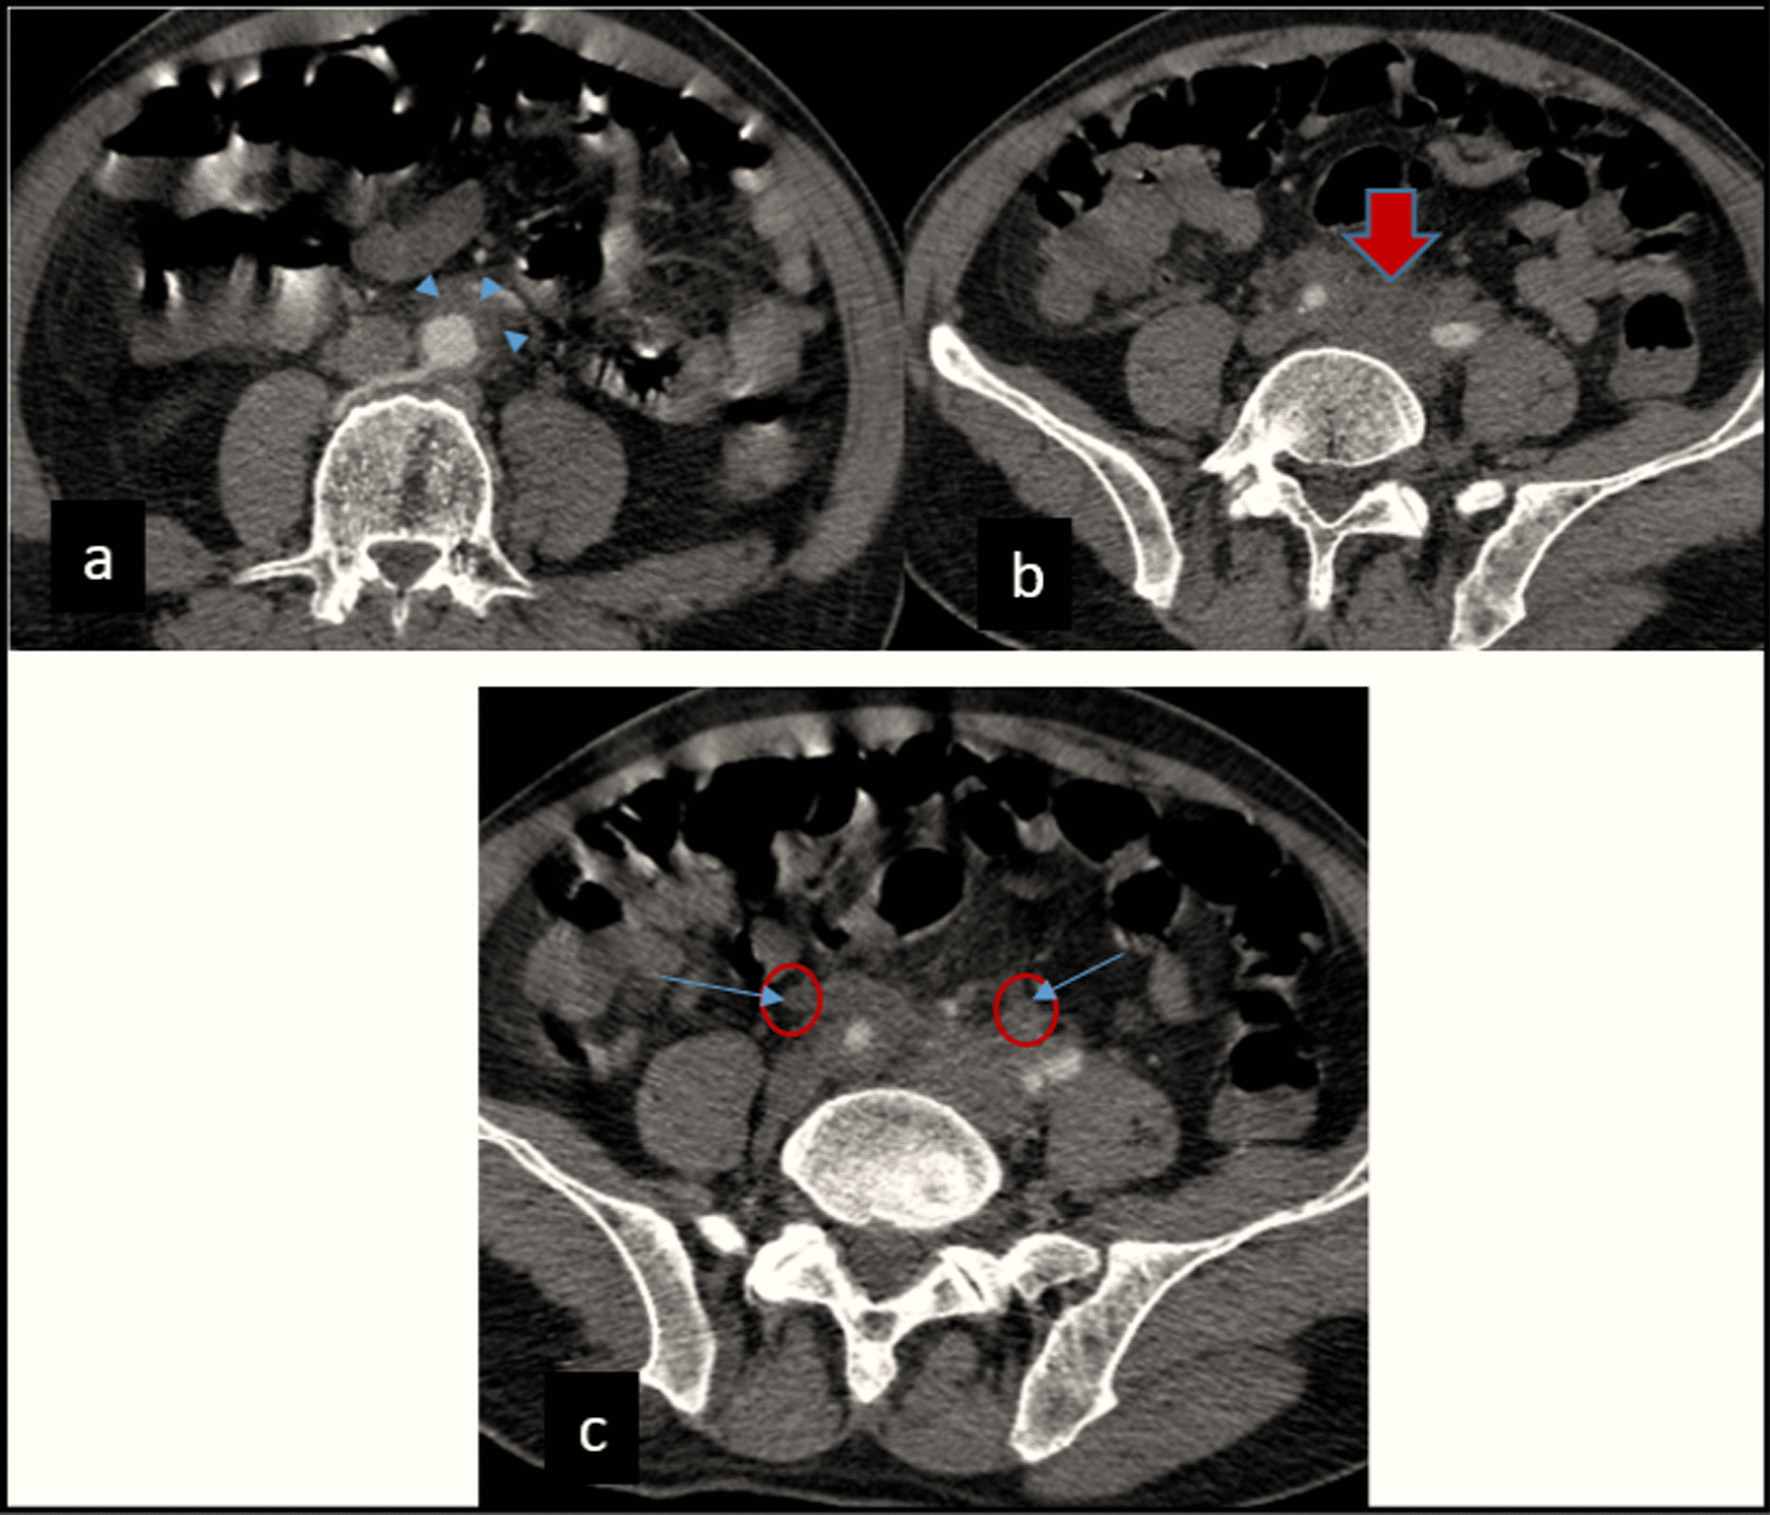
Abdominal CT scan, axial section:; tissue density infiltrate (or sleeve), homogeneous, perivascular, sheathing the abdominal aorta (a, arrowheads), extending to the iliac arteries (b, red arrow) and iliac ureters (c, blue arrows) in favor of retroperitoneal fibrosis (RFP)
